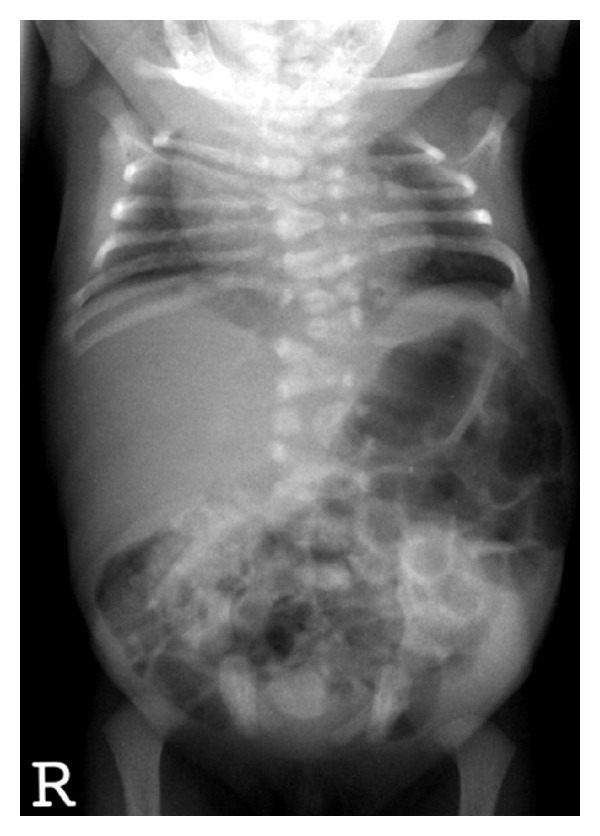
Radiographic features of spondylocostal dystostosis including contiguous vertebral malformations with asymmetric rib malformations. Photograph courtesy of Peter D. Turnpenny M.D., Royal Devon and Exeter Hospital.
